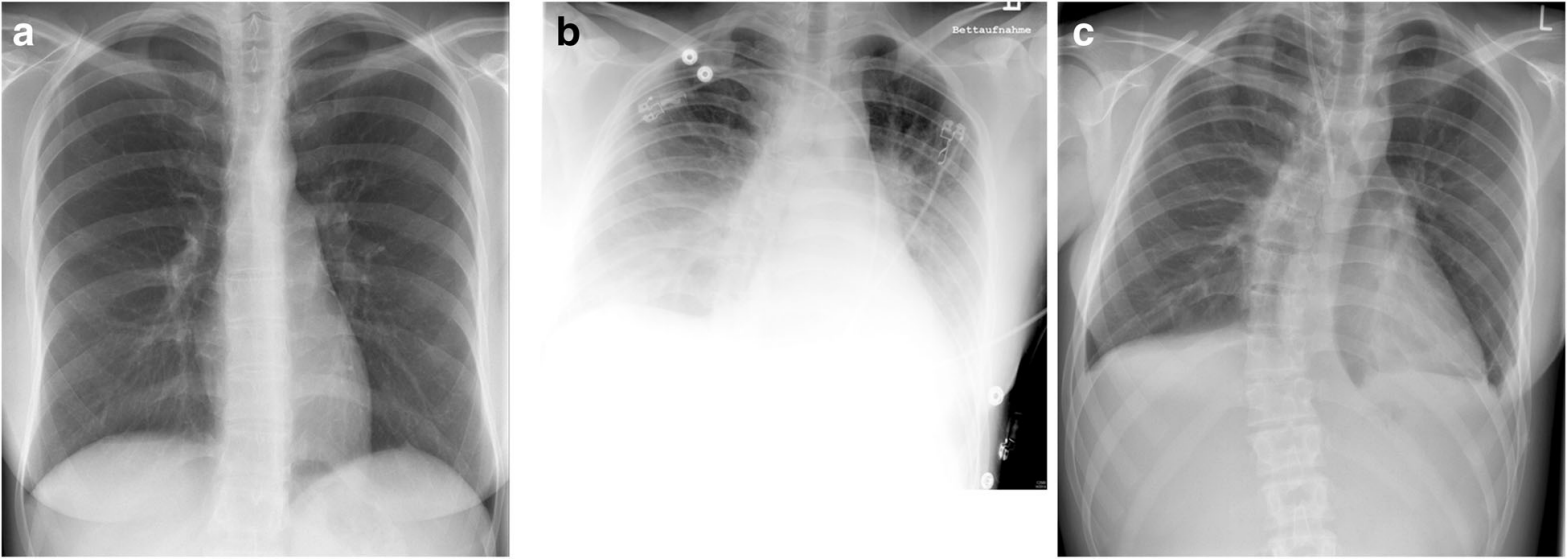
Chest x-ray of patients infected with PUUV (a, admission) and DOBV-Sochi (b, admission, bedside chest x-ray; c, after renal replacement therapy, 12 dpo)
